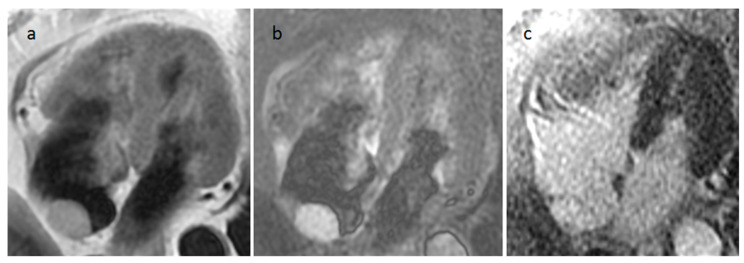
Right atrial myxoma isointense on T1-weighted images (a), hyperintense on T2-weighted images (b), hyperintense and mildly heterogeneous on late gadolinium enhancement sequence (c).
